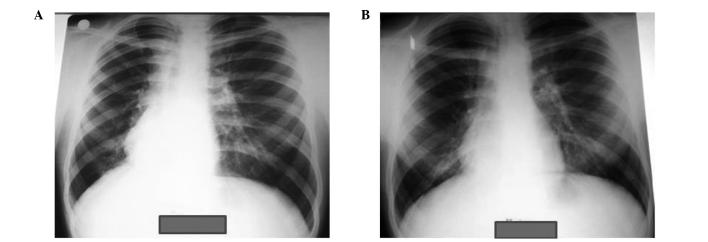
Chest X-rays of a 14-year-old male with a positive tuberculin skin test induration of 16 mm (BCG-vaccinated) and dextrocardia, (A) prior to and (B) following preventative treatment. Pneumonic infiltration was detected at the follow-up in the right lower zone of the chest X-ray after 50 days of isoniazid treatment.
